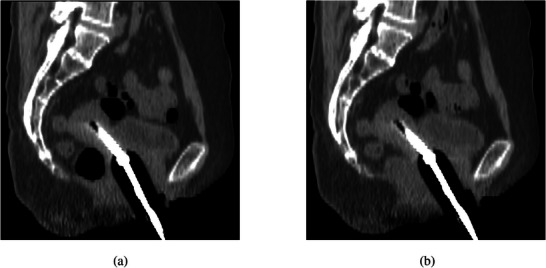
Representative CT images before and after rectal gas evacuation (a): pre‐evacuation; (b): post‐evacuation).
